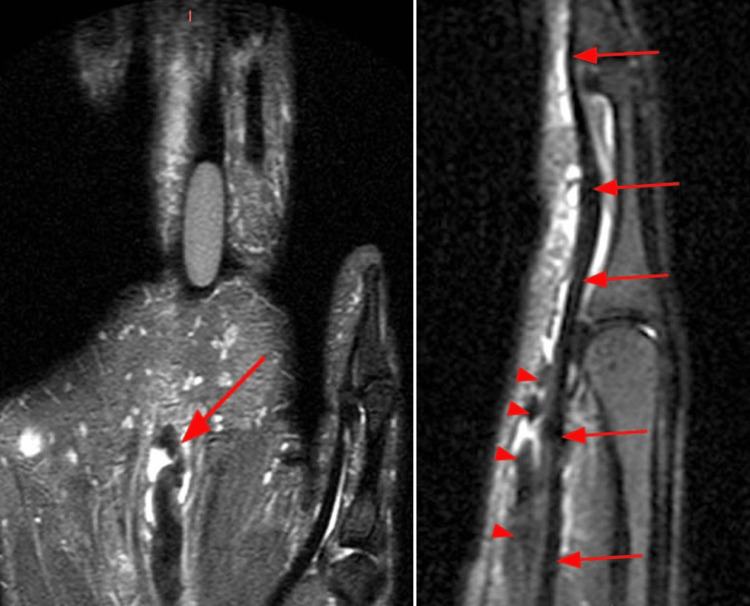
MRI images of the left hand MRI images clearly demonstrate the intact FDP tendon (arrows) found deep to the proximally retracted and redundant FDS, compatible with an isolated closed rupture. FDP: Flexor digitorum profundus, FDS: Flexor digitorum superficialis
